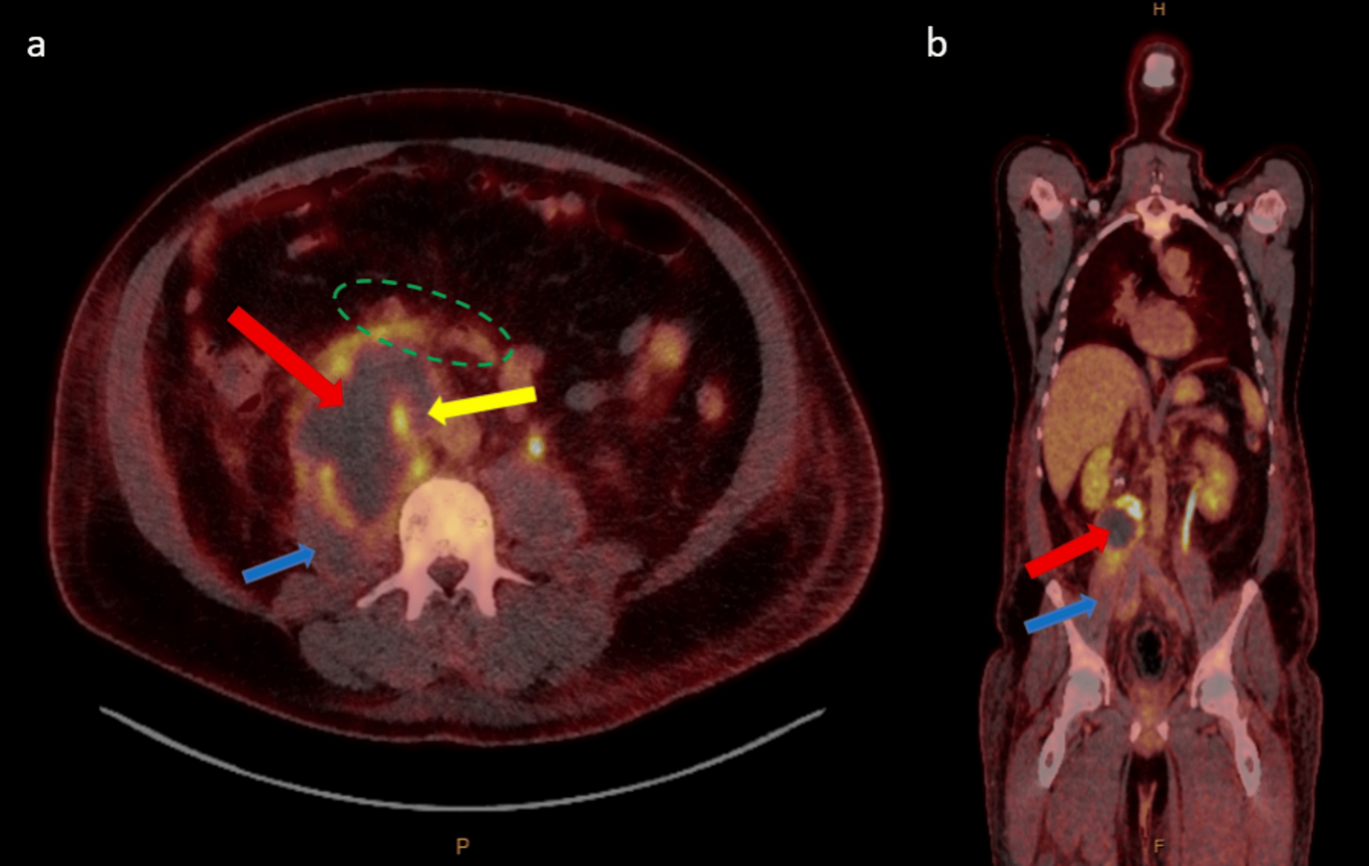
a-b showing fused PET-CT phase pre-operatively PET: positron emission tomography (PET); CT: computed tomography; IVC: inferior vena cava (a) Axial slice demonstrating a right retroperitoneal mass (red arrow) with central photopenia and peripheral moderate to high-grade increased activity extending to the right psoas muscle (blue arrow), duodenum (green dotted oval), and IVC (yellow arrow). (b) Coronal slice demonstrating right retroperitoneal mass (red arrow) with activity extending to the right psoas muscle (blue arrow)
